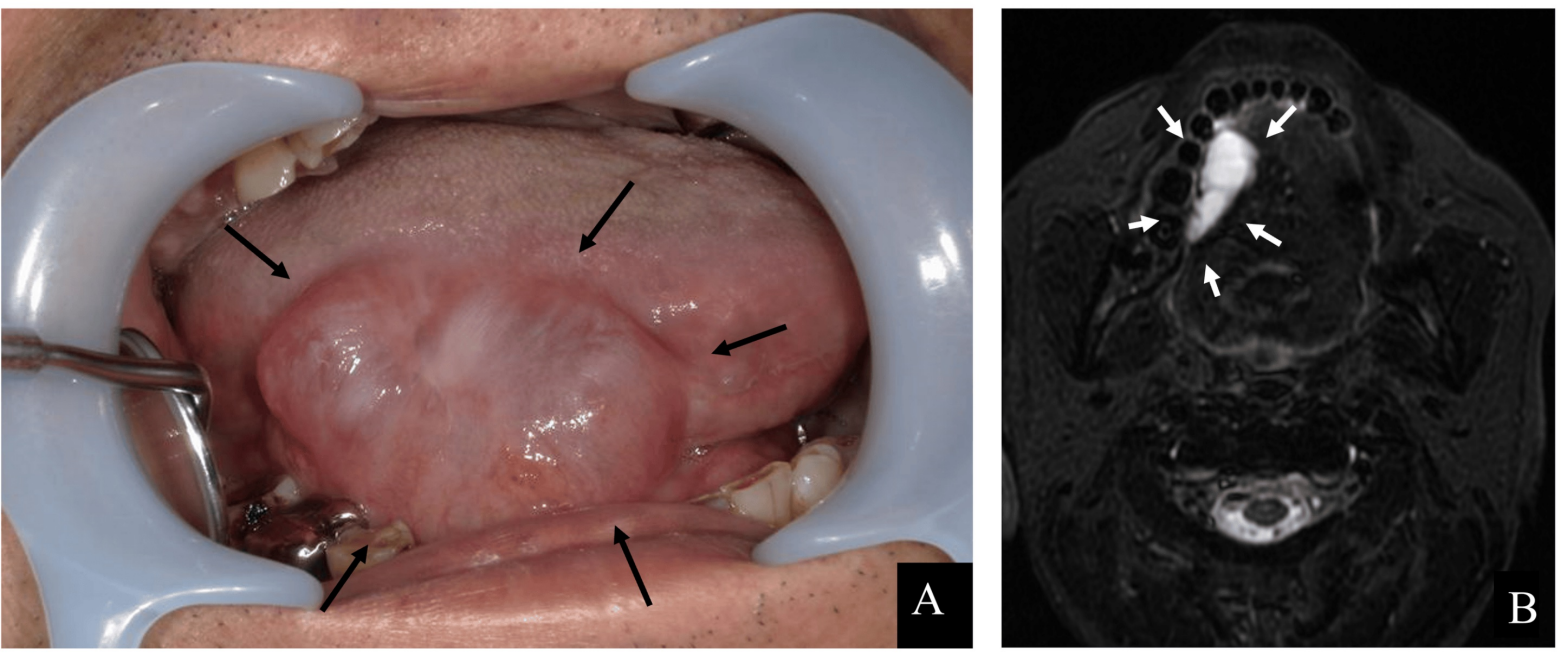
Intraoral photographs and MRI of ranula that appeared seven months after tongue cancer surgery. (A) An intraoral photograph was taken eight months after the initial visit (seven months after tongue cancer surgery). A 38 x 26 mm blue-purple mass was seen at the right lingual border. (B) Contrast-enhanced MRI, T2-weighted image was taken eight months after the initial visit (seven months after tongue cancer surgery). A cystic high-signal area suggestive of fluid retention was observed in the right sublingual crevice.
